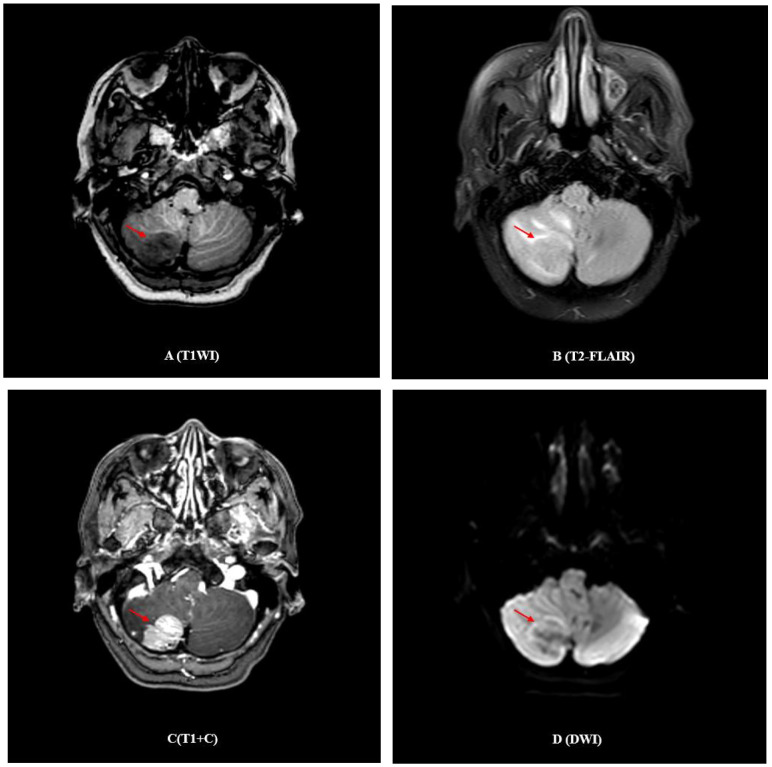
Brain MRI of the cerebellar metastasis. (A) T1-weighted imaging shows a hypointense lesion. (B) T2-FLAIR imaging demonstrates extensive peritumoral edema (hyperintense signal). (C) Post-contrast T1-weighted imaging reveals heterogeneous marked enhancement. (D) Diffusion-weighted imaging shows no significant diffusion restriction. The lesion measures 2.3 × 2.7 cm. The patient refused standard therapy and was followed with serial imaging.
